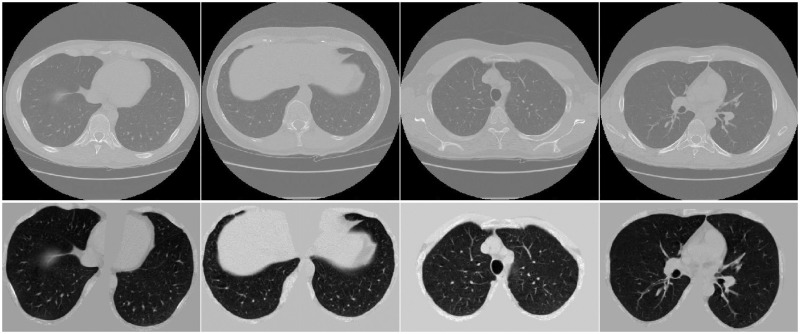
CT images before and after preprocessing. The CT images in Fig 4 were accessed through the links available on this page: https://luna16.grand-challenge.org/Download/ and had not been previously published and/or copyrighted.
